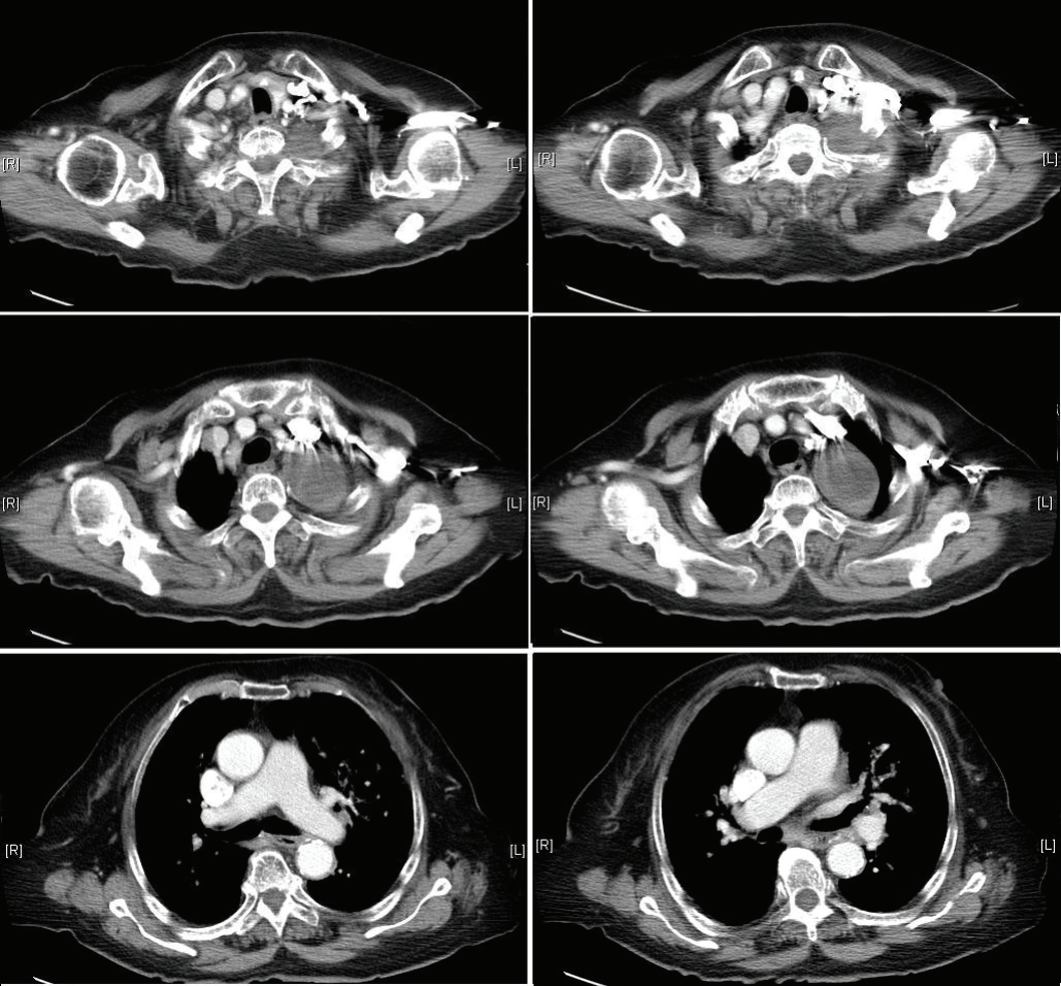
Chest CT (with contrast) demonstrates a 4.0 cm soft tissue mass at left lung apex compressing the left common carotid artery without compromise of blood flow. There are several oval lymph nodes at mediastinum.
